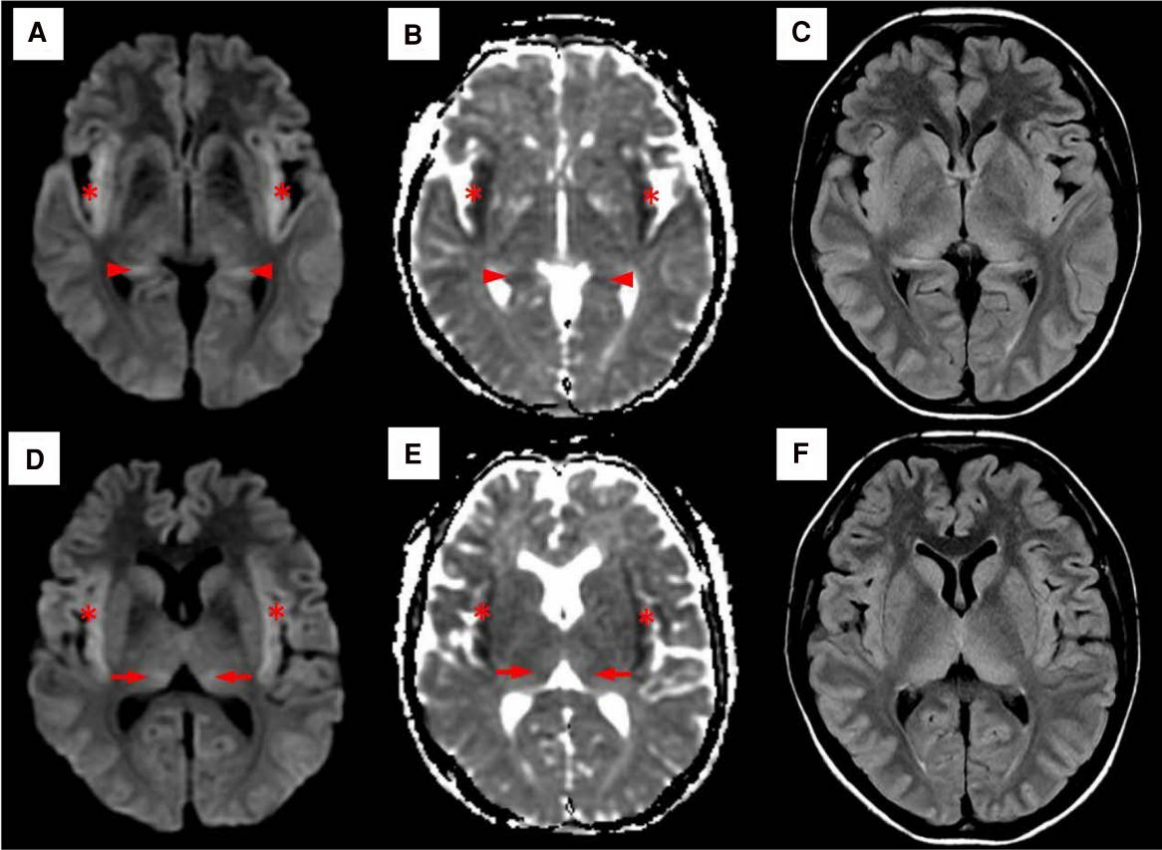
Axial brain magnetic resonance imaging showed bilateral symmetrical diffusion-weighted imaging hyperintense (*A* and *D*) and apparent diffusion coefficient hypointense (*B* and *E*) signal abnormalities in the bilateral insula (*), medial thalami (arrows), and hippocampi (arrowheads), consistent with restricted diffusion in these areas. These regions also showed corresponding symmetrical T2 fluid-attenuated inversion recovery hyperintensities (*C* and *F*).
